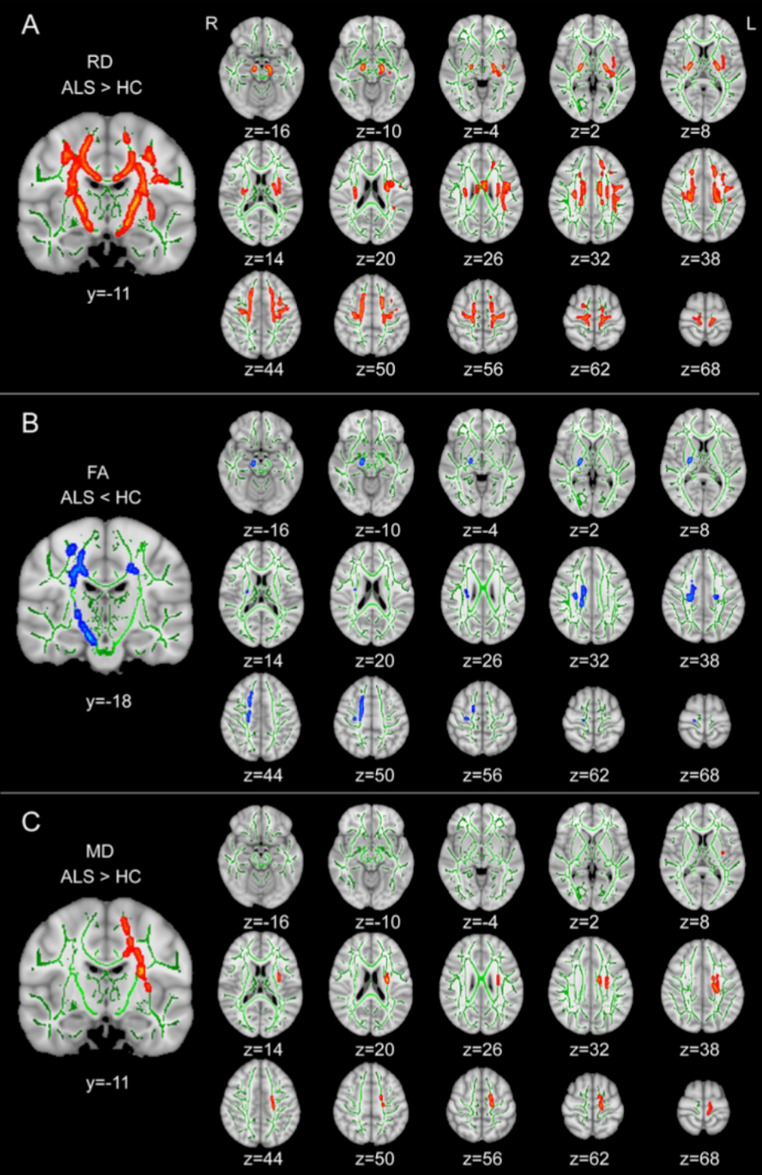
Degree of WM microstructural involvement in ALS. Results of a TBSS analysis showing the degree of WM microstructural changes occurring in in ALS patients, with altered RD, FA and MD values across the whole brain (WM skeleton in green). Regions in yellow/red scale indicate a significant increase in RD (upper panel, A) and MD (lower panel, C), while blue-light blue scale indicate significant decrease in FA values (middle panel, B). Reprinted with permissions from [112]. ALS: amyotrophic lateral sclerosis; WM: white matter; dMRI: diffusion magnetic resonance imaging; TBSS: tract-based spatial statistics; RD: radial diffusivity FA: fractional anisotropy; MD: mean diffusivity
